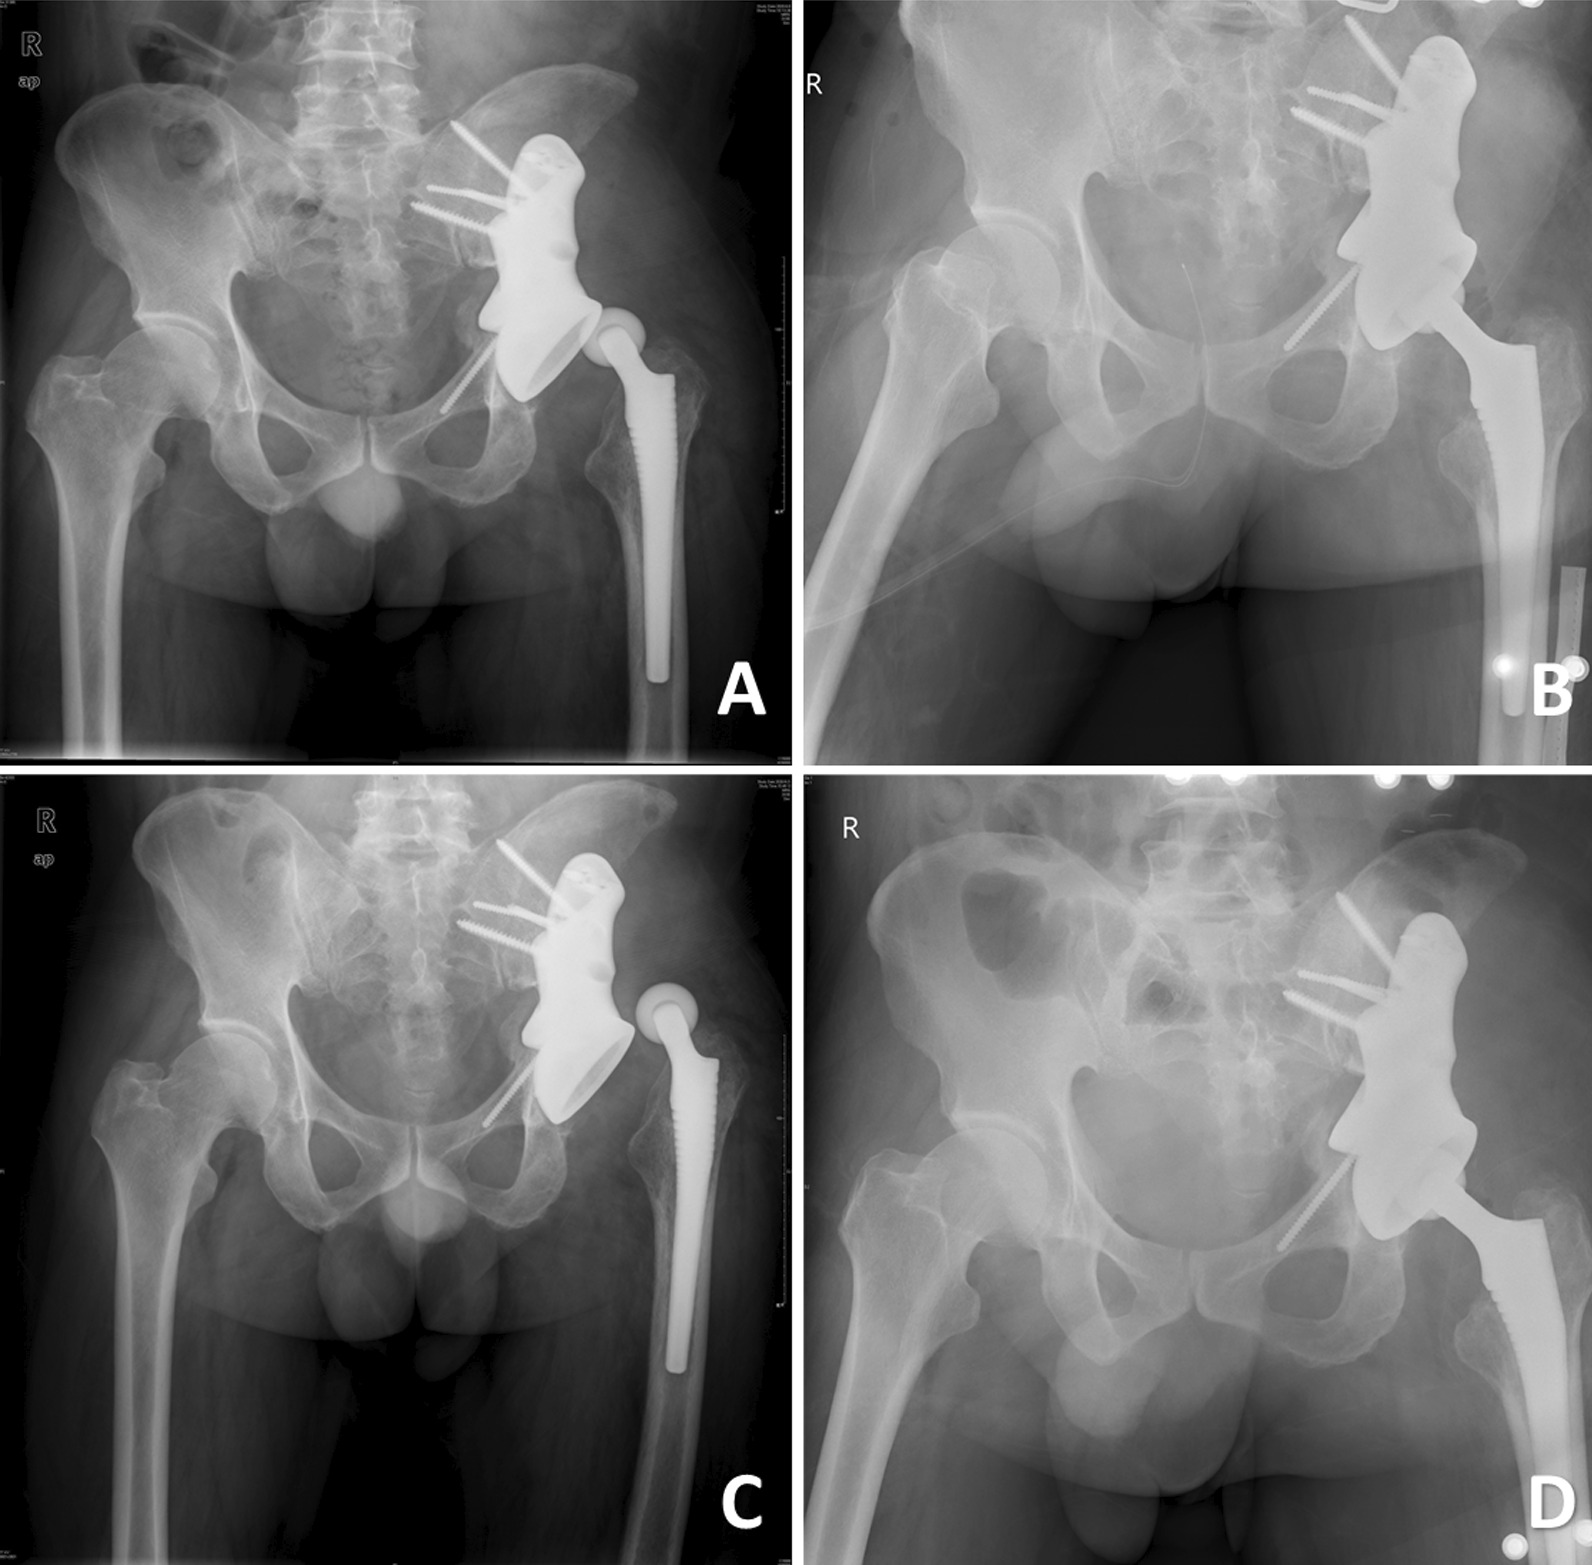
Radiograph images of a patient with recurrent dislocation. A Radiograph image obtained before the open reduction. B Radiograph image obtained after the reduction. C Radiograph image obtained before placement of the polyethylene liner and femoral head. D Radiograph image obtained after placement of polyethylene liner and femoral head
